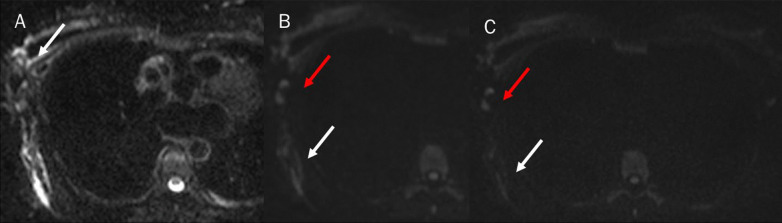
Magnetic resonance study: diffusion weighted imaging (DWI) sequences in axial plane (A: b50 s/mm^2^; B: b500 s/mm^2^ and C: b800 s/mm^2^). White arrows show breast and chest lymphangitis (A–C). Red arrows show lymphadenopathy (A–C)
